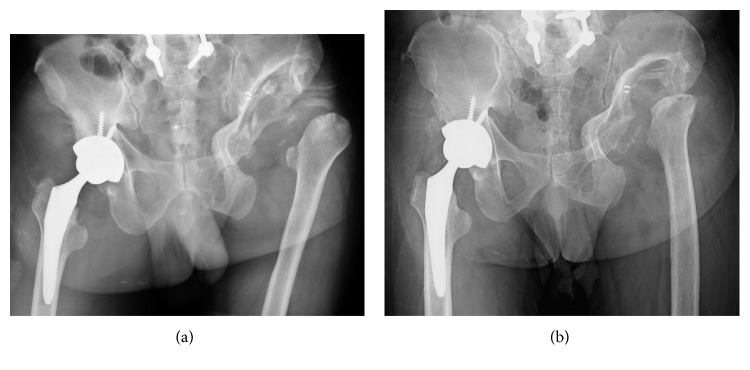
Case 2 postoperative hip X-rays. (a) Immediately after operation. (b) Five years after operation. There were no clinical signs of implant loosening in the hip X-ray.
